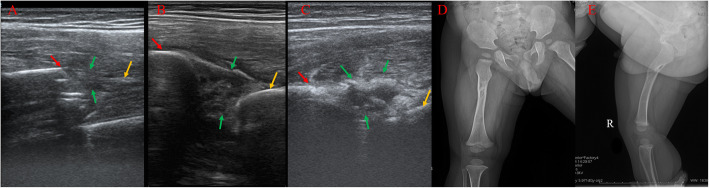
Images of a 2-year-old boy with FSF. The red and yellow arrows show the distal and proximal fractures, respectively. The green arrow shows the callus. a Ultrasound imaging on day 3 after injury, during the hematoma period. b Ultrasound imaging on day 8 after the injury, during the organization of the hematoma, which could be adjusted and reduced. This could not be visualized by radiographs (green arrow). c The appearance of the callus indicating the stabilization of the fracture, which can be seen on the radiographs. d, e Radiographs showing the anteroposterior and lateral views during the final follow-up
